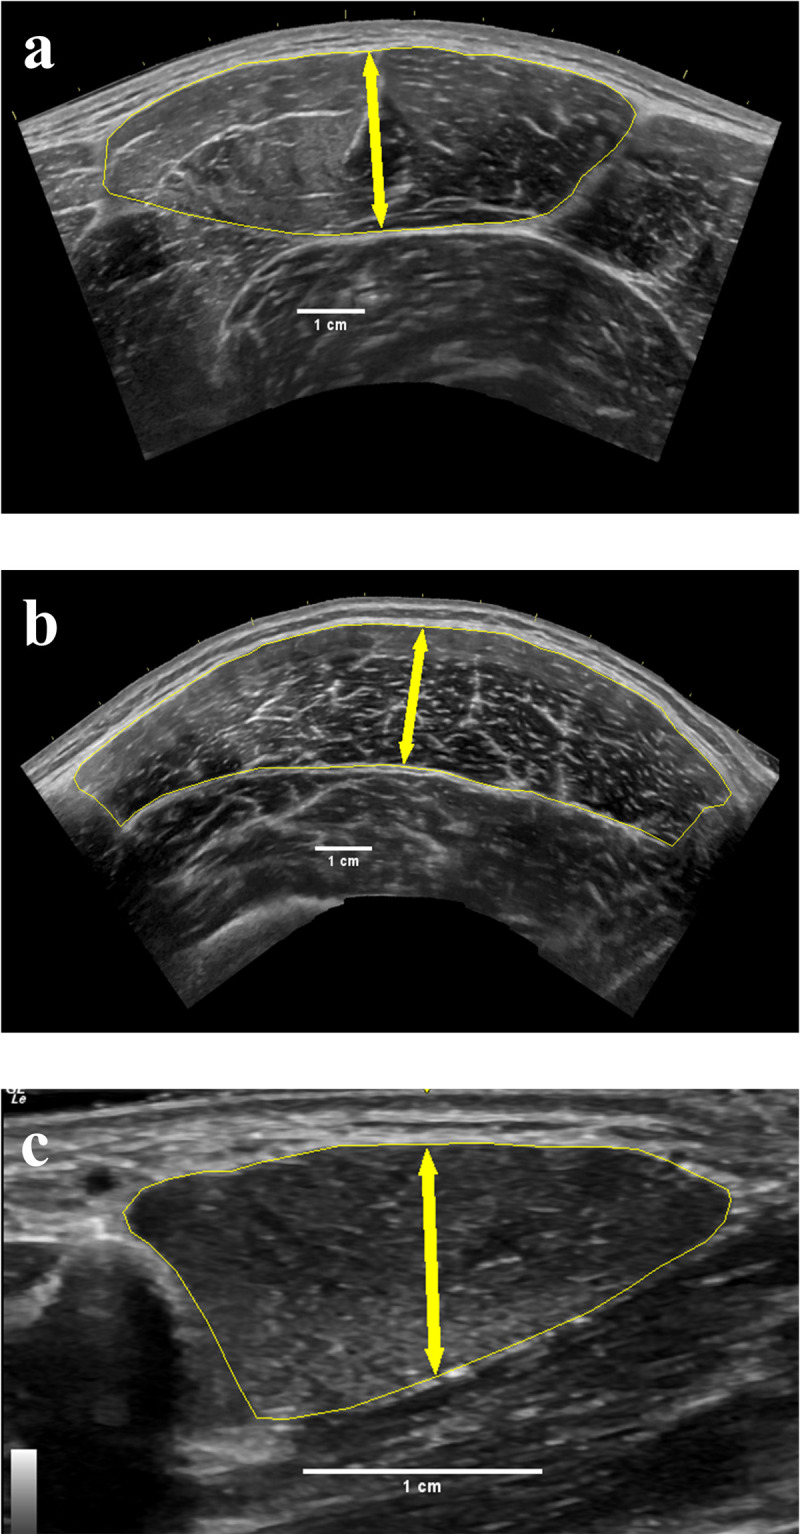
Ultrasound-derived images of the three skeletal muscles utilized in the ImageJ analyses; a = rectus femoris; b = vastus lateralis; c = first dorsal interosseus. The yellow outline represents CSA while the double-sided arrow represents muscle thickness.
